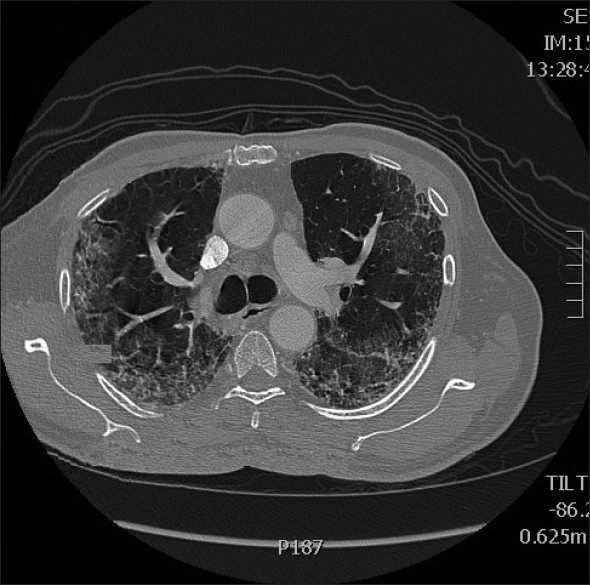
High resolution computed tomography scan 2
